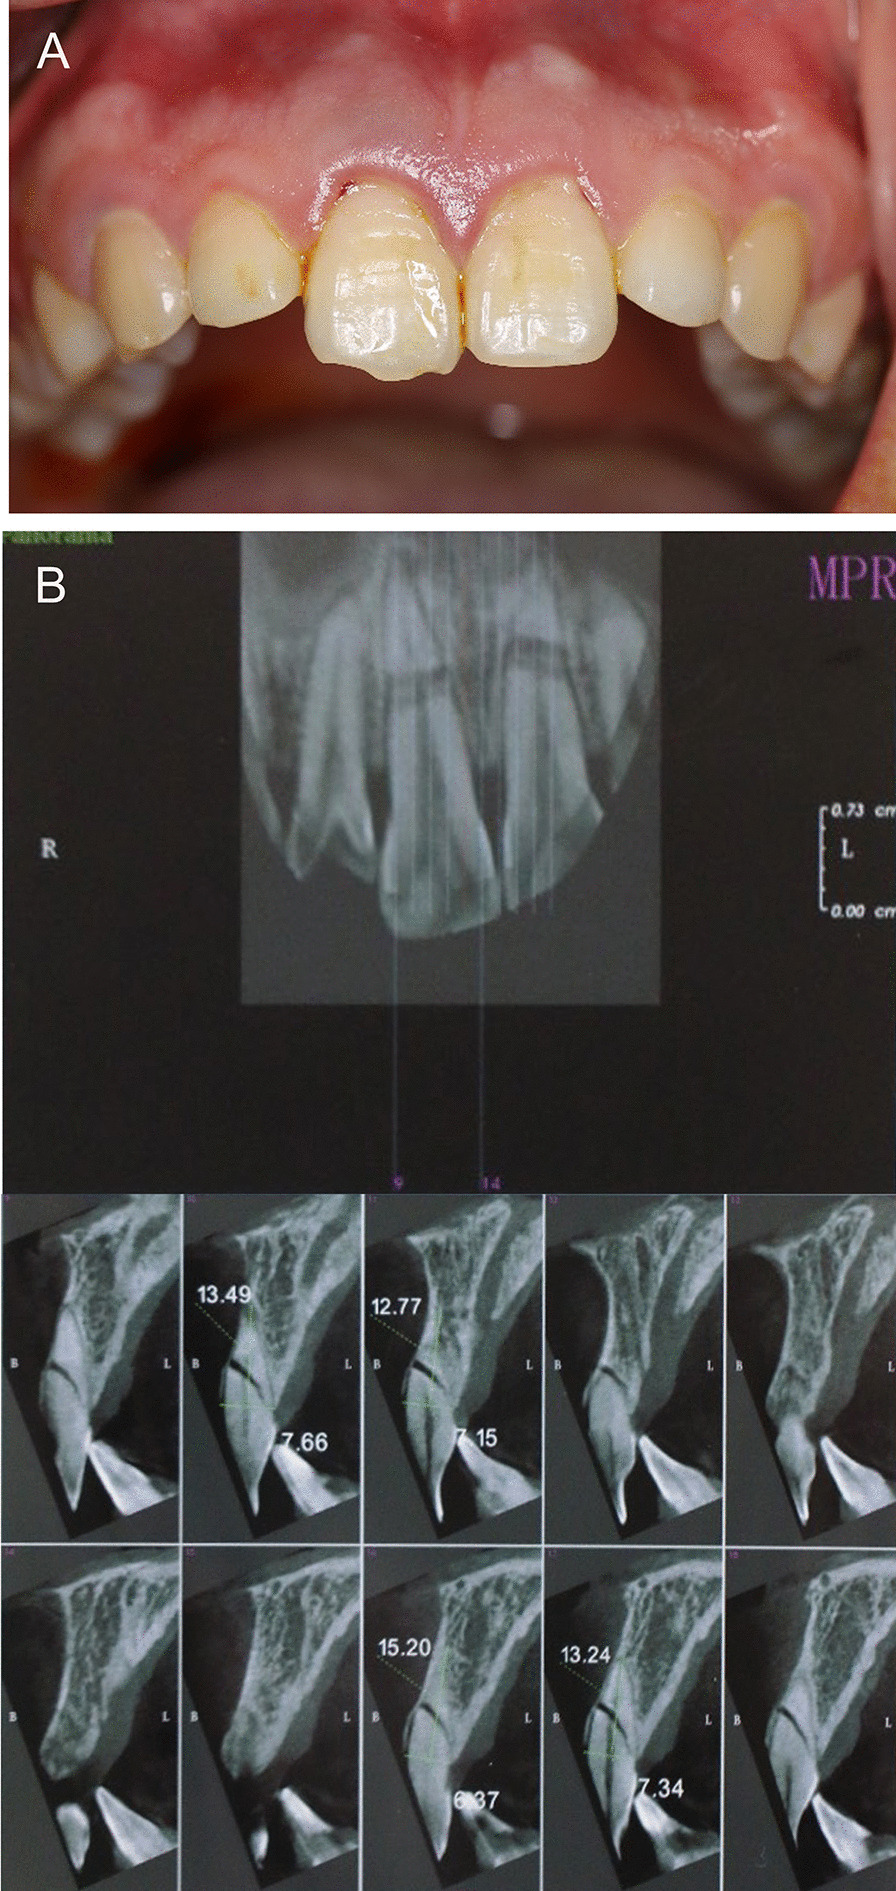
Preoperative view. A Intraoral photograph, B preoperative CBCT
